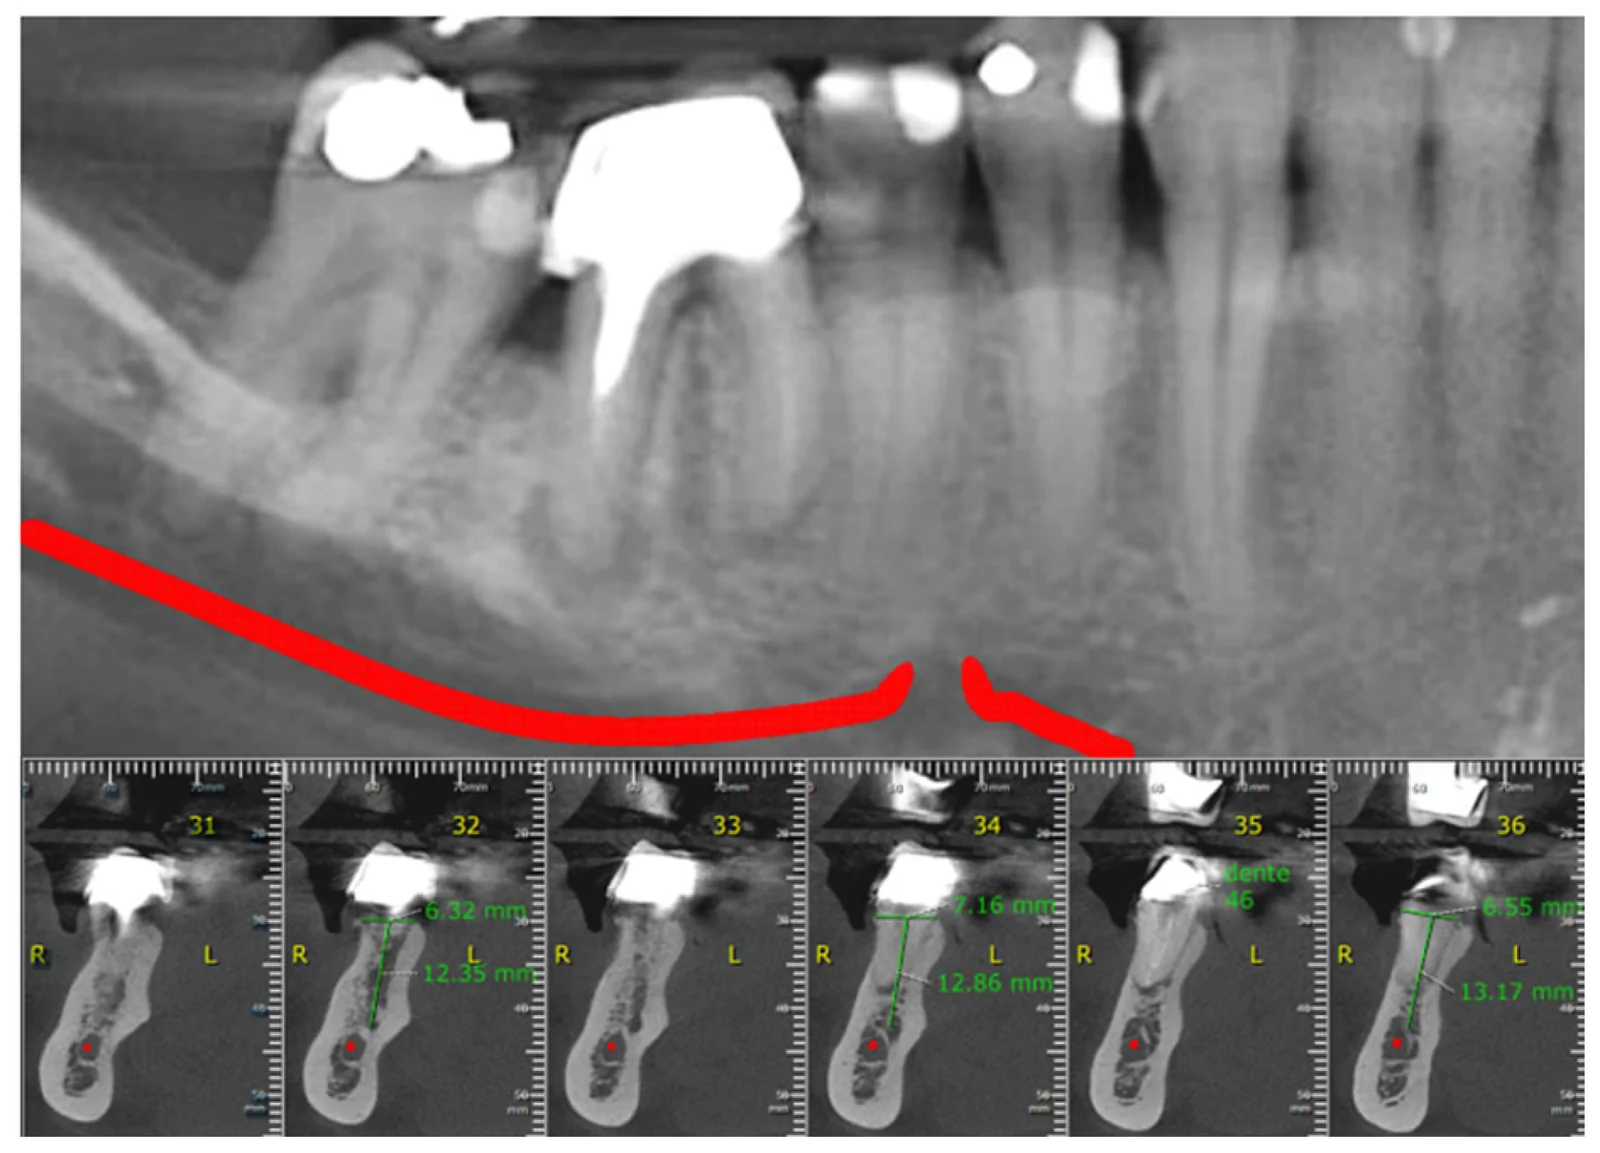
Baseline cone-beam computed tomography.
